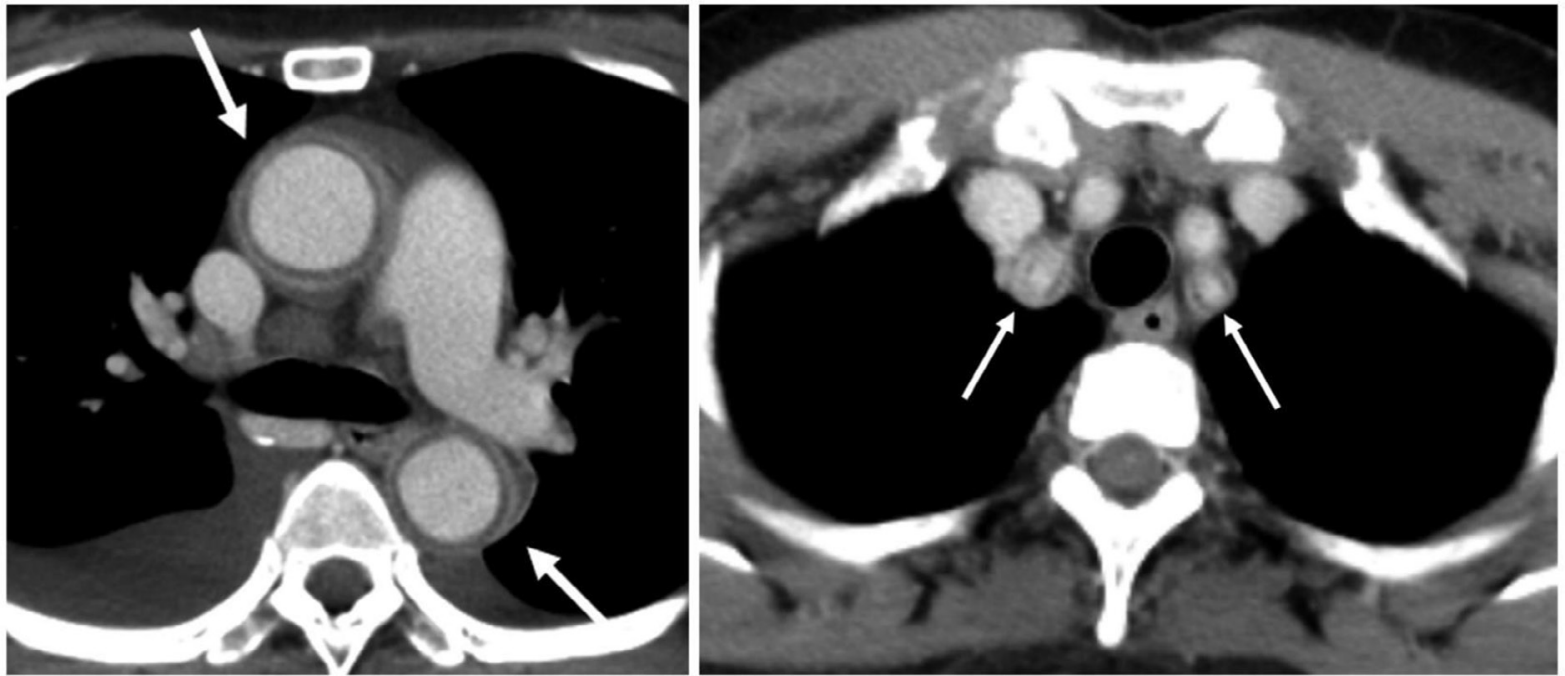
Early phase axial CT scan demonstrating typical concentric wall thickening with double rings, note that the inner ring is hypodense compared to the relatively hyperdense outer ring. The image on the left shows involvement of the ascending and descending aorta while the image on the right demonstrates involvement of the bilateral proximal subclavian arteries.
